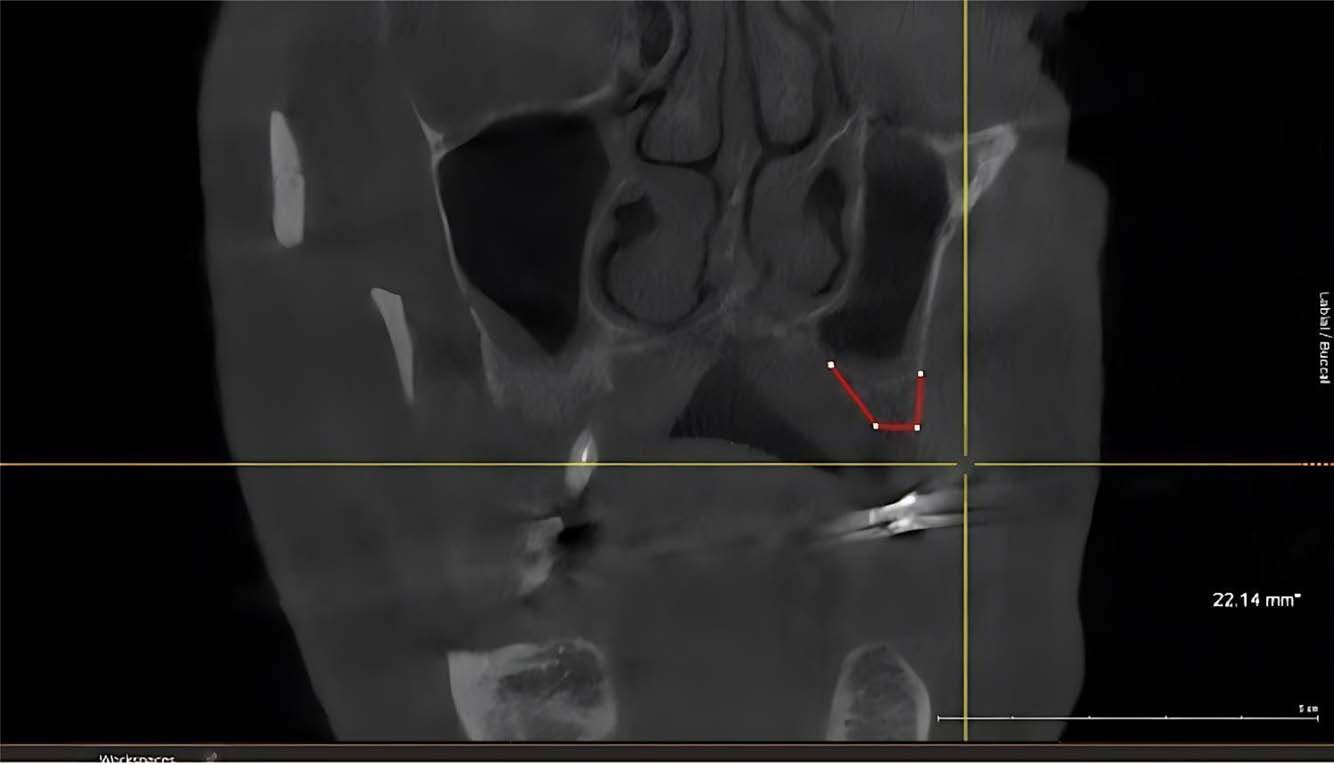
CBCT as Siebert’s classification bone defects at maxillary left class II for female patient. CBCT = cone beam computed tomography.
